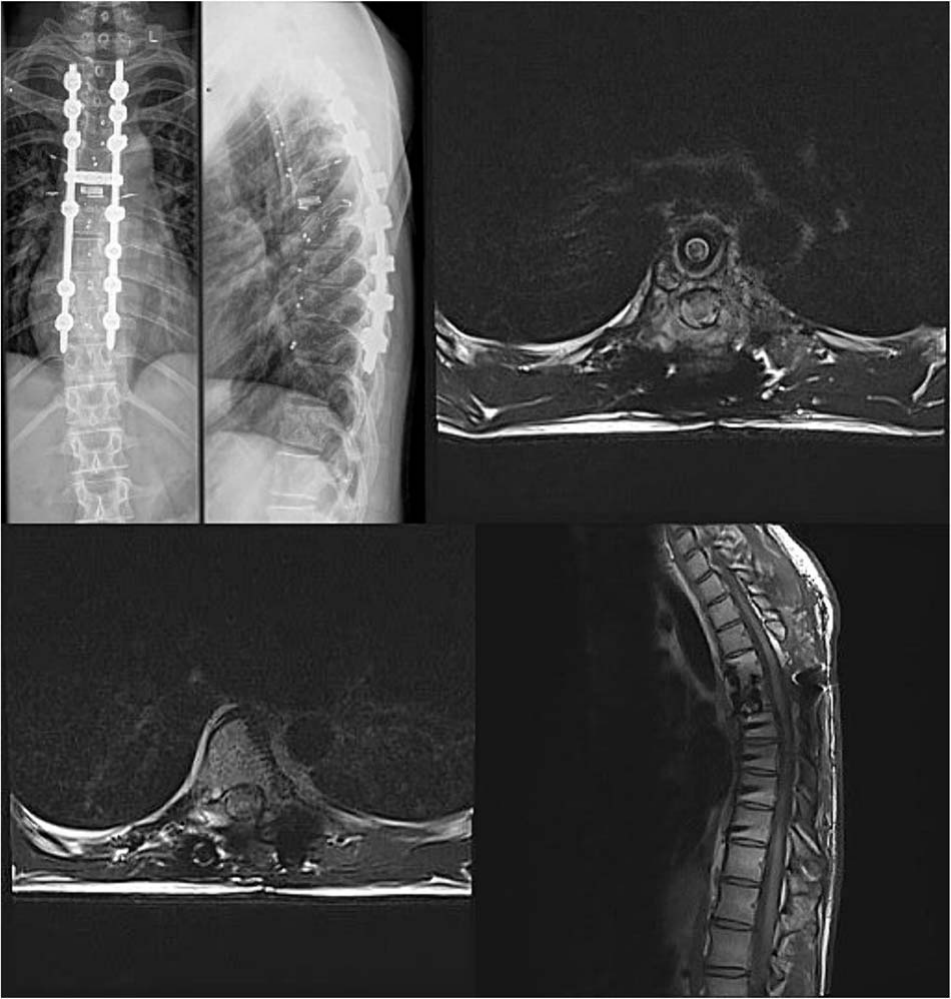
Final follow-up X-Ray scan and MRI imaging showing no radiological signs of spinal instability or myelopathy.
